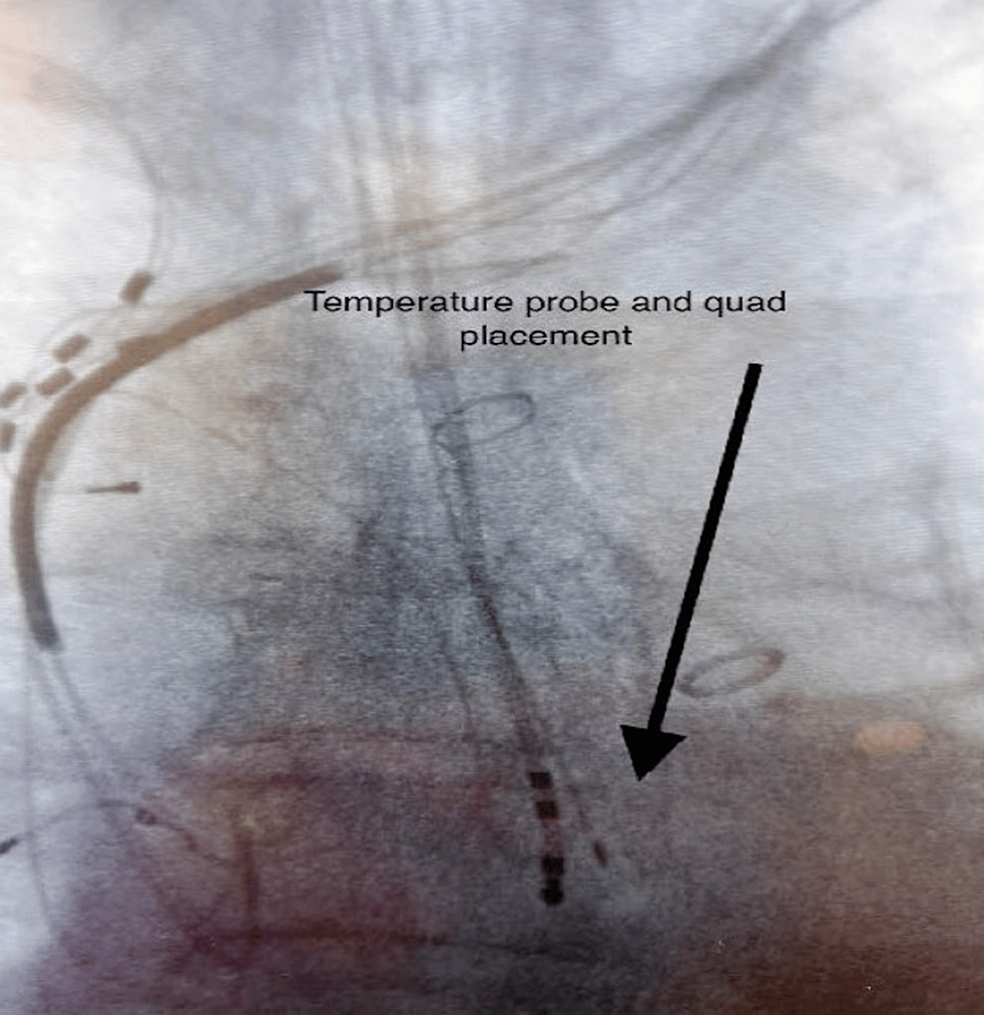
Placement of the CIRCA S-CATH M™ esophageal temperature probe under fluoroscopy. The placement of the esophageal temperature probe is confirmed using either fluoroscopy (as shown) or using transesophageal echocardiography (TEE). The position is usually confirmed using TEE in our clinical practice.
